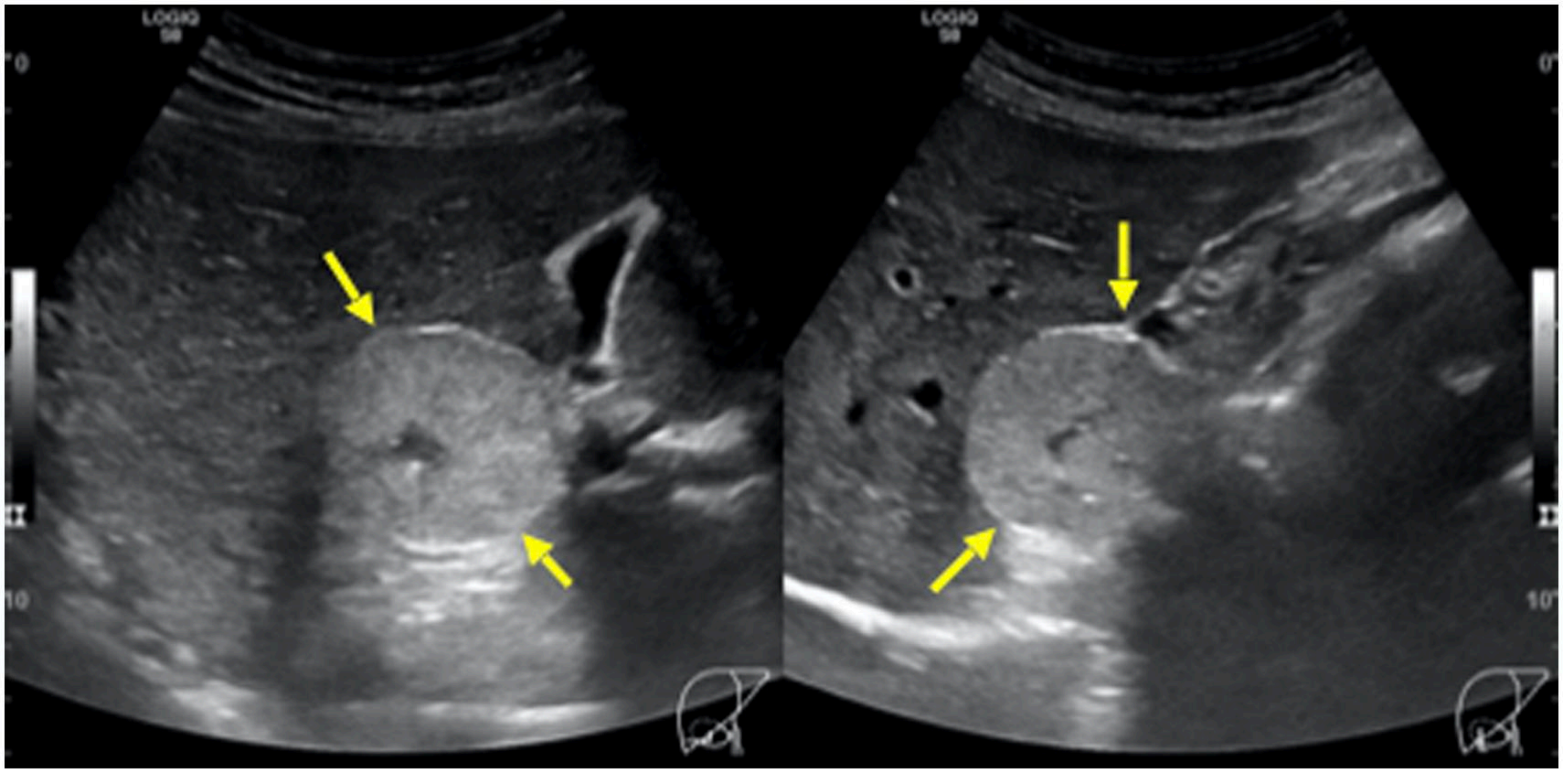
Abdominal ultrasound in axial and sagittal planes at the right upper quadrant reveals a hyperechoic mass with clearly defined margins (yellow arrows), adjacent to the inferior right hepatic lobe.
